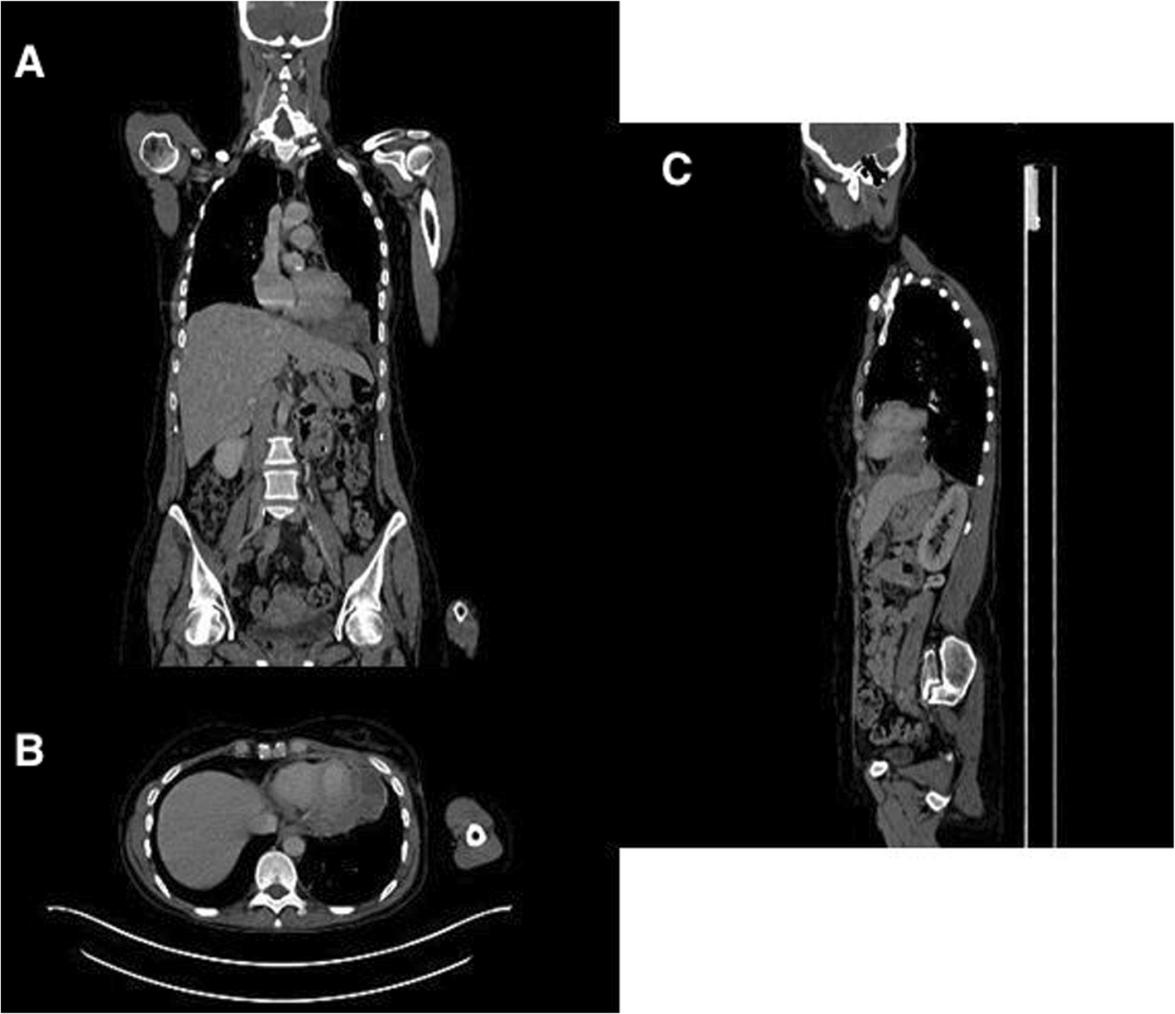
Coronal (a), transverse (b), and sagittal (c) computed tomographic images of pericardial mass
